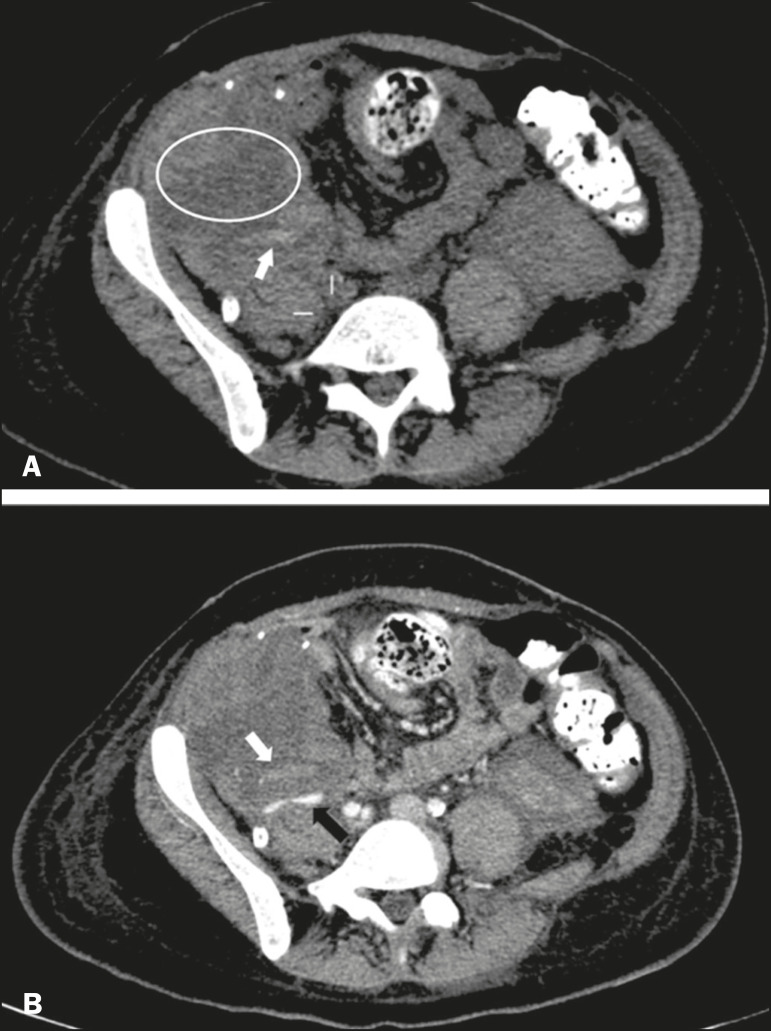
A: Unenhanced abdominal CT scan in patient who underwent SPKT, showing a linear image of a spontaneously hyperattenuating mass (arrow) suggestive of thrombosis. In addition, the pancreatic graft is heterogeneous, with hypoattenuating areas (ellipse). B: Contrast-enhanced abdominal CT scan, in the venous phase, of the same patient, showing arterial opacification (black arrow) and the absence of venous opacification (white arrow), confirming the venous thrombosis seen in the previous phases.
